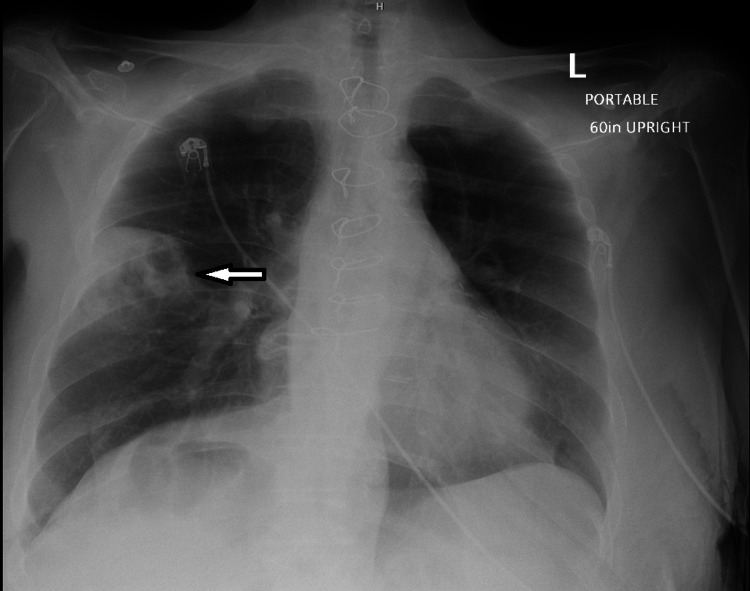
Chest radiograph obtained at initial admission demonstrating right middle lobe opacity.
